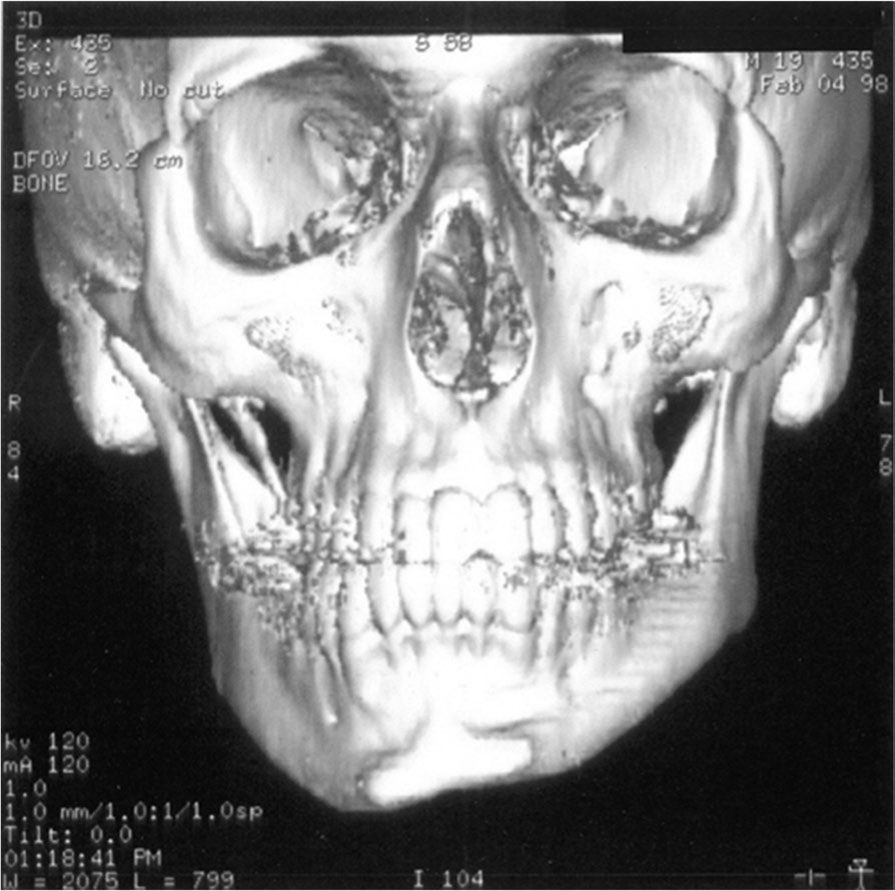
Computed tomography (CT) with 3D reconstruction. The facial asymmetry is due to the underdevelopment of the left side of the mandible
